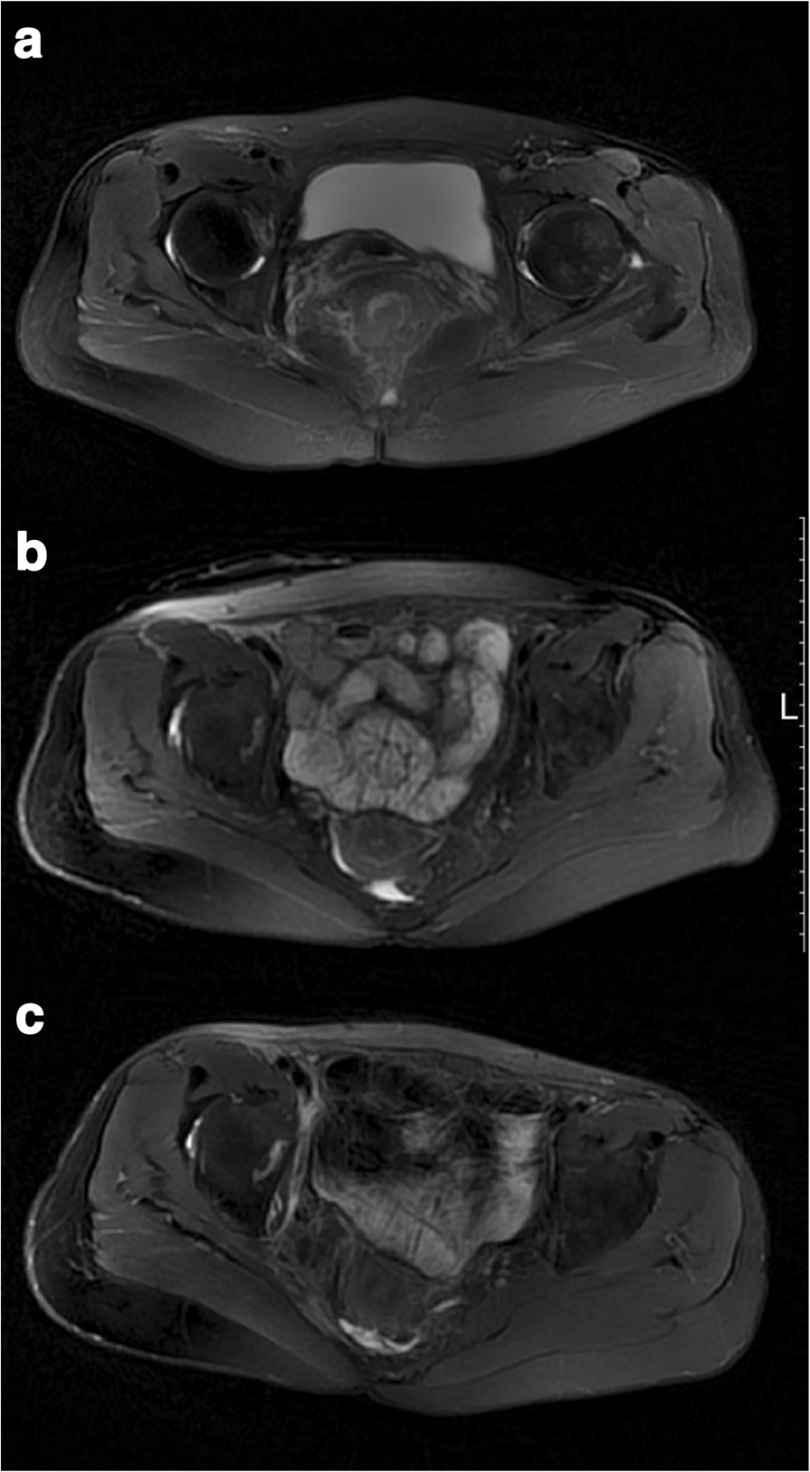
The Pelvic MRI images. a Preoperative image. b Postoperative 9th month image. c Postoperative 24th month image
